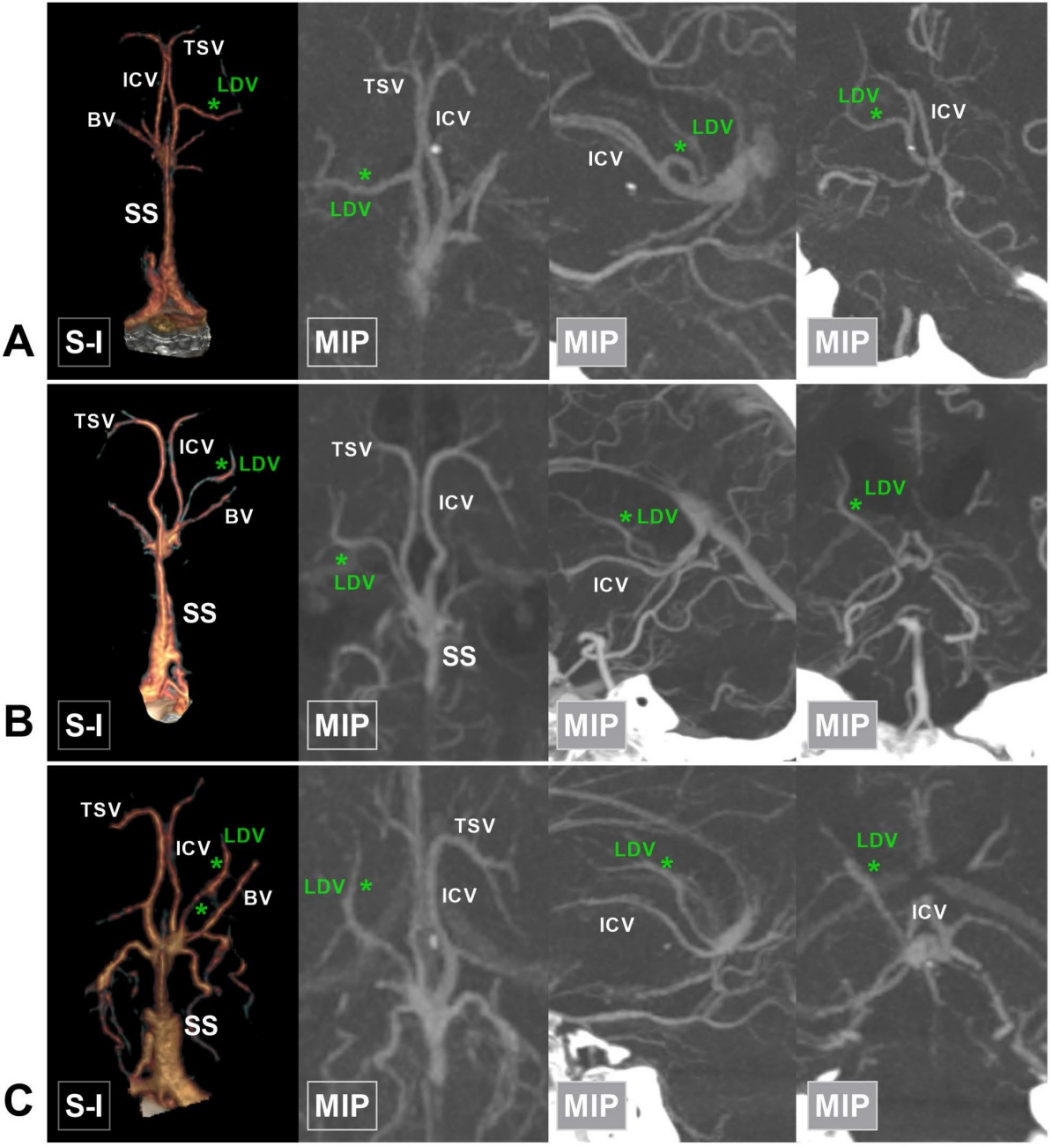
The posterior type of LDV. A, CTA and MIP show that the LDV (asterisk) drains into the middle of the ICV and extends laterally. B, CTA and MIP show that the LDV (asterisk) drains into the posterior half of the ICV. C, CTA and MIP show that the LDV (asterisk) drains into the confluence of the ICV and BV. Abbreviations: BV, basal vein; CTA, computed tomography angiography; ICV, internal cerebral vein; LDV, lateral direct vein; MIP, maximum intensity projection; SS, straight sinus; TSV, thalamostriate vein.
